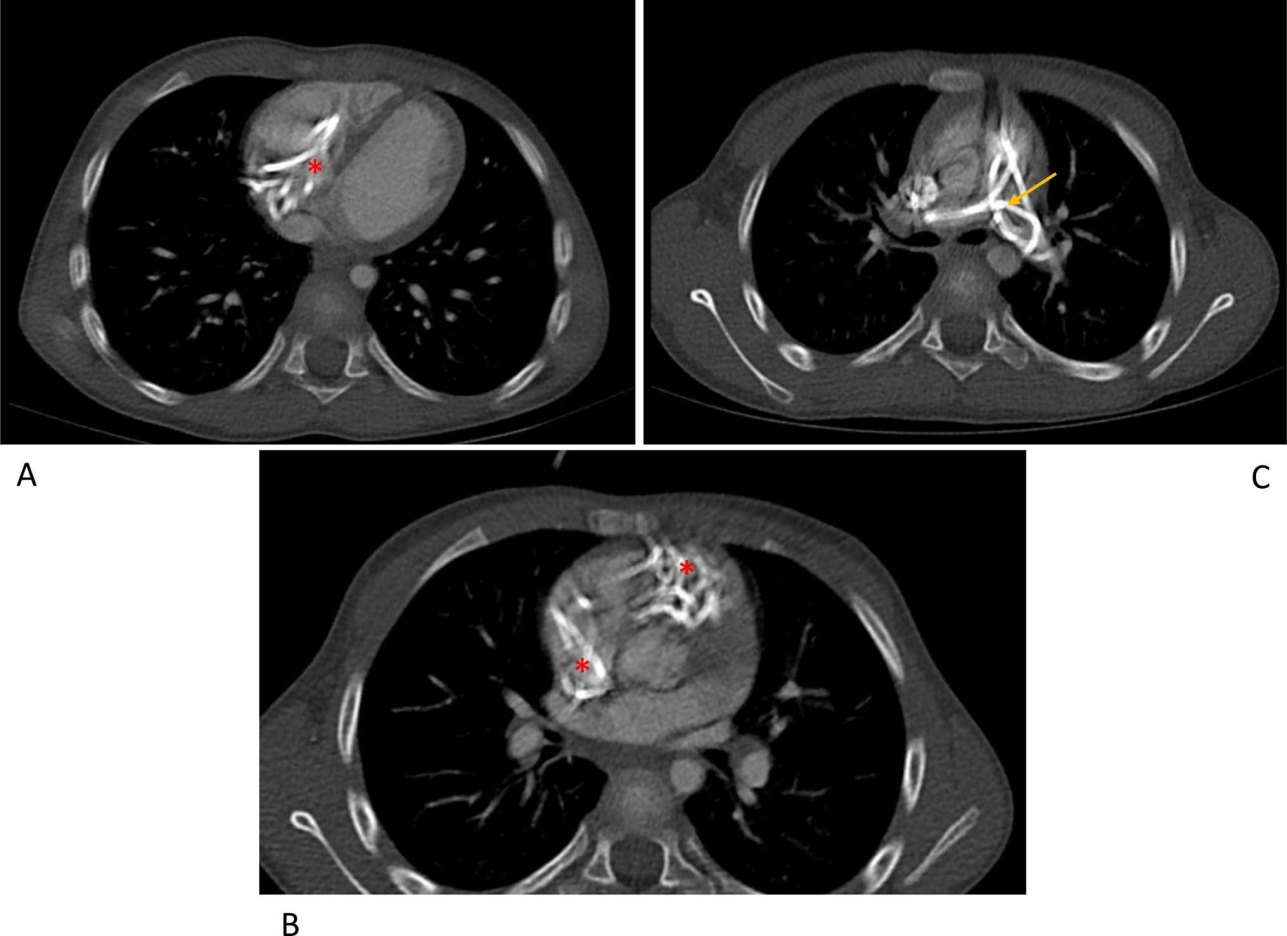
Axial thoracic CT scan images demonstrating extent of shunt coiling and knotting within the atrium and ventricles (A, B) (red stars) and pulmonary arteries (C) (yellow arrow) indicating the significant complication risks of removing the distal shunt via direct extraction
